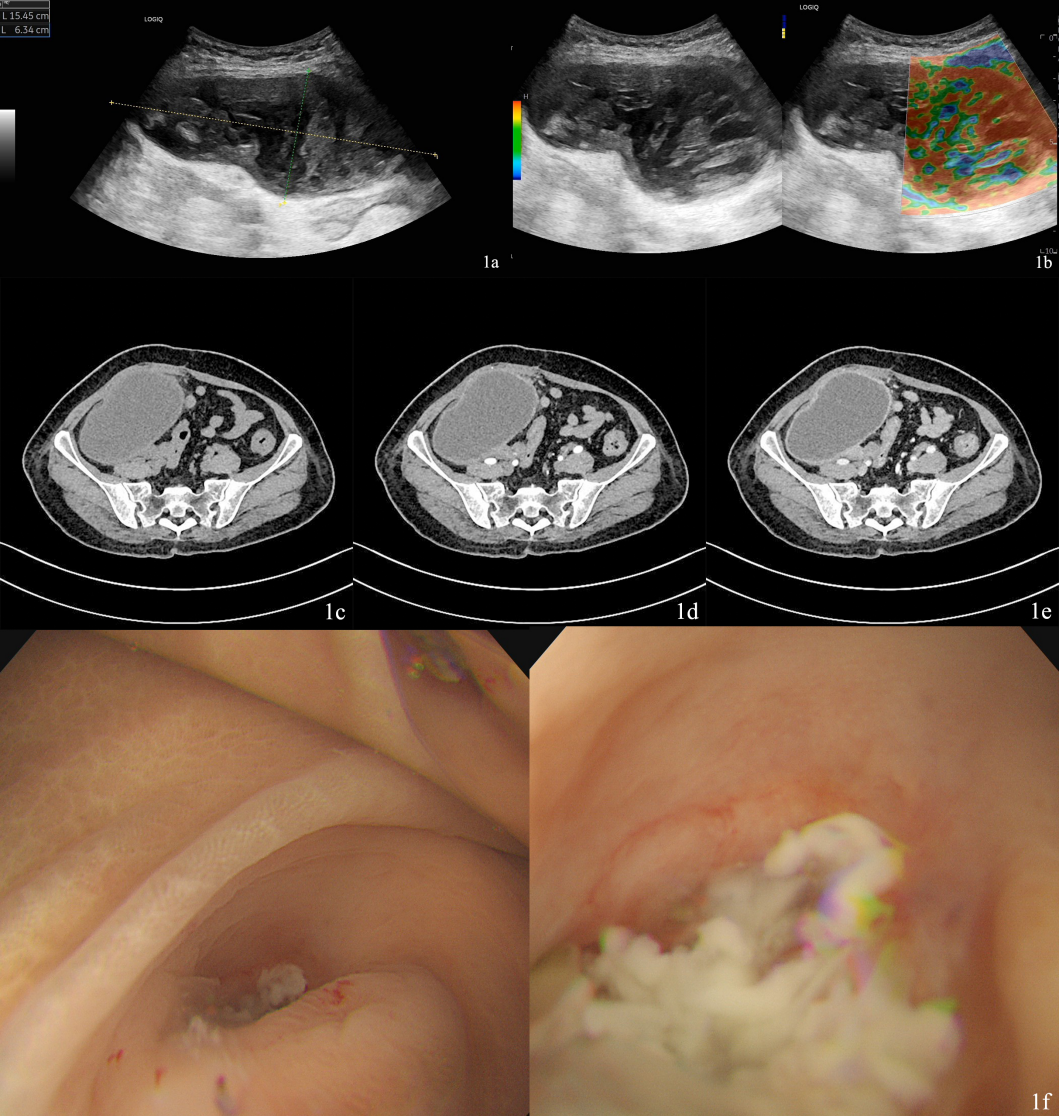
(A) Ultrasound showed a cystic and solid mixed mass approximately 15.4×6.3cm in size. (B) No intratumoral. Blood flow signals were detected. (C) The mass exhibits a well-defined capsule and hypodense internal contents. (D) Contrast-enhanced CT showed marked wall enhancement without internal vascularity. (E) Contrast. Enhanced CT showed marked wall enhancement without internal vascularity. (F) Colonoscopy showed white mucous discharge was observed at the appendiceal orifice.
